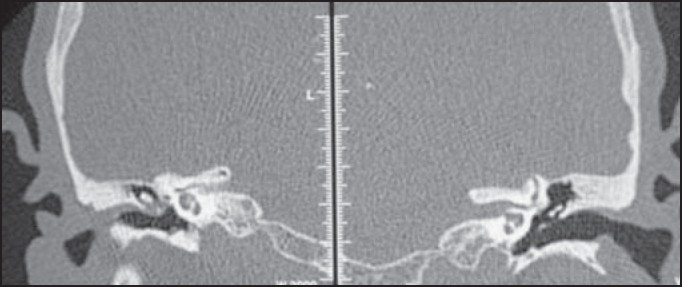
Temporal bone CT scan at coronal section evidencing partial obliteration of right middle ear and oval window niche with soft part density materials.
